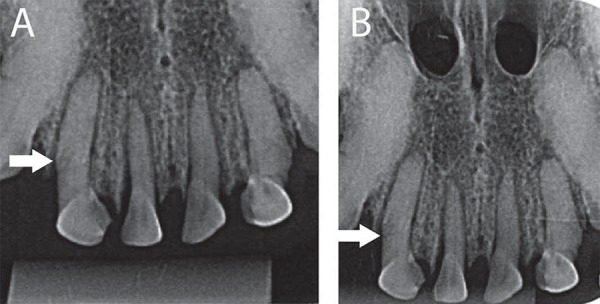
A: Periapical radiograph of the right lateral incisor, showing root fracture (filled arrow). Orthogonal angulation; B: Periapical radiograph of the right lateral incisor, demonstrating root fracture (filled arrow). 10º vertical angulation from orthogonal
